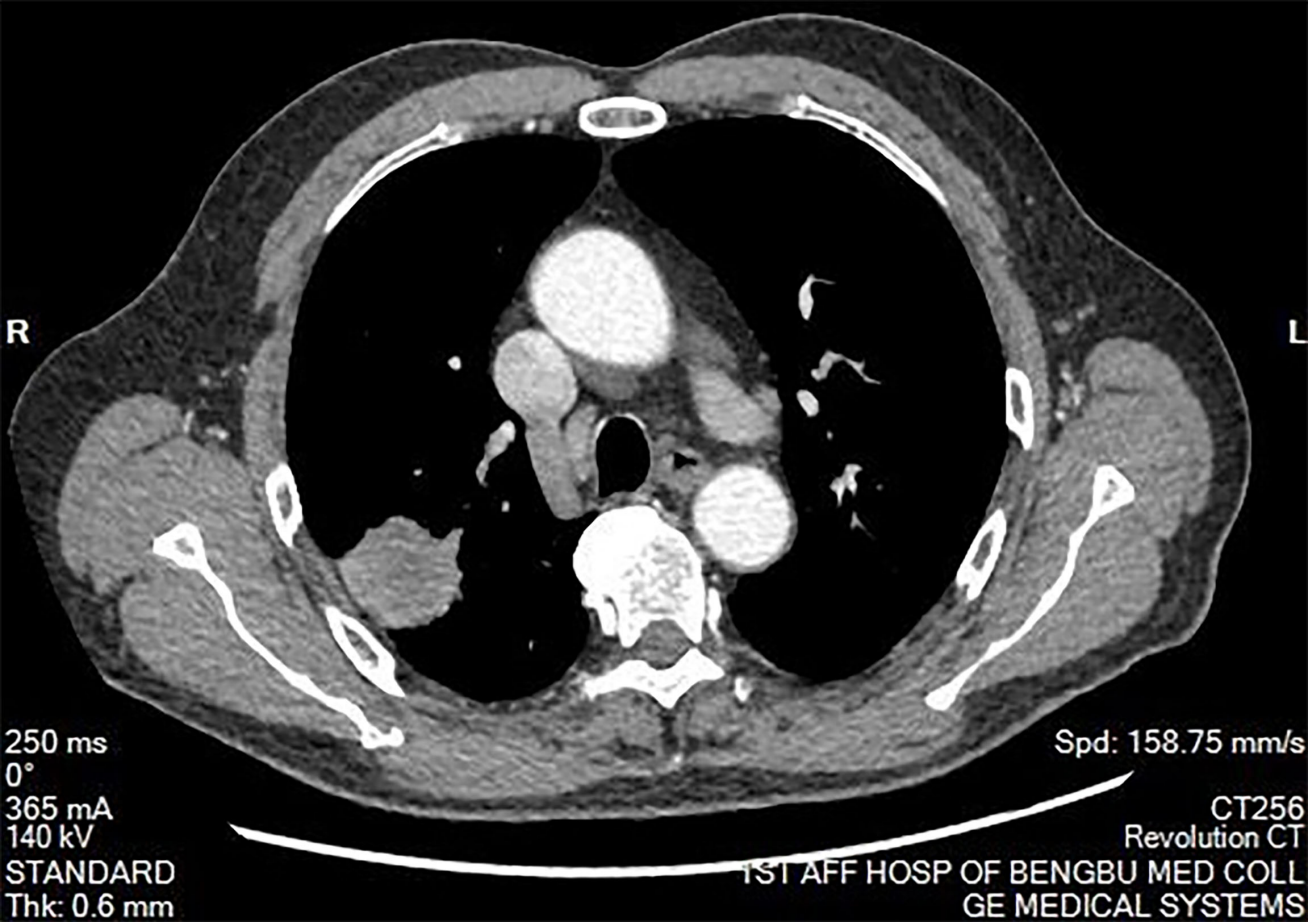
Mediastinal window of chest computed tomography. There was no abnormal mass in the mediastinum. The hilum on both sides is normal. There was no pleural effusion on both sides.
